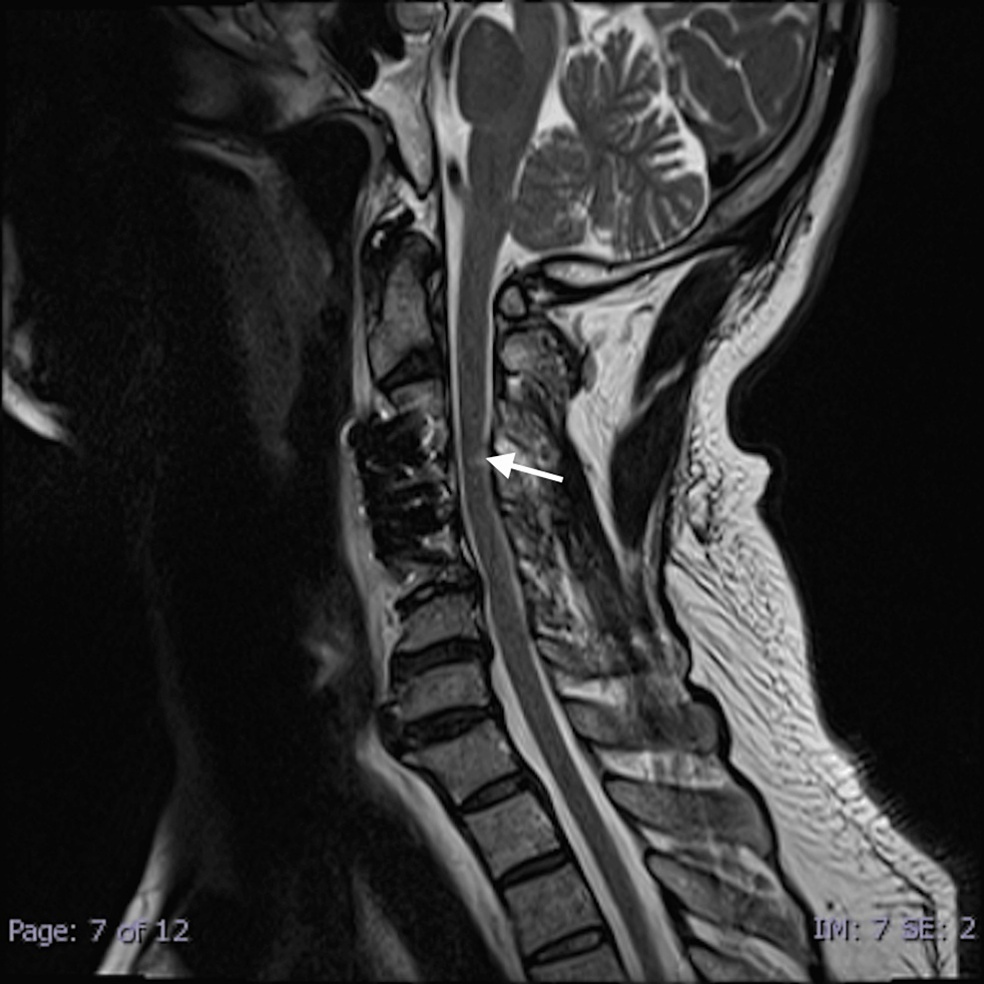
Sagittal T2-Weighted Image Sagittal T2-Weighted MR image of the spine shows a hyperintense signal change on the spinal cord, indicative of white cord syndrome.
